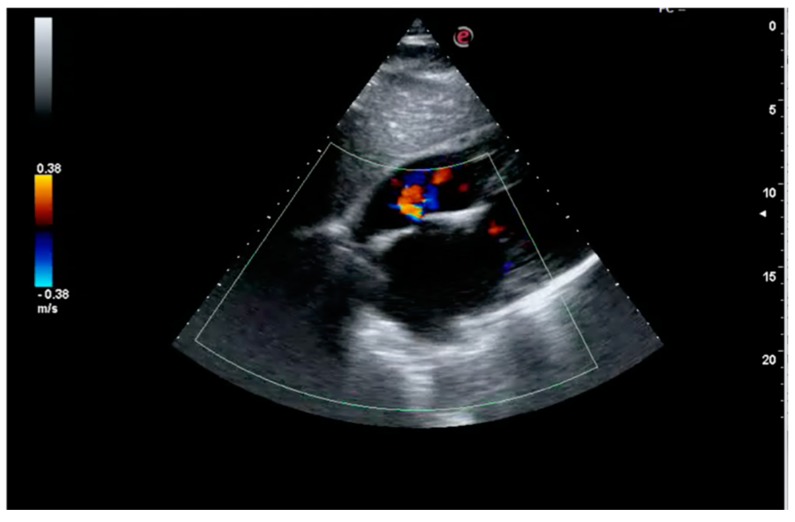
Transthoracic echocardiogram (subxiphoid view). The exam showed a clearly discernable interatrial septal defect with left-to-right shunt identified, at rest, with color doppler.
